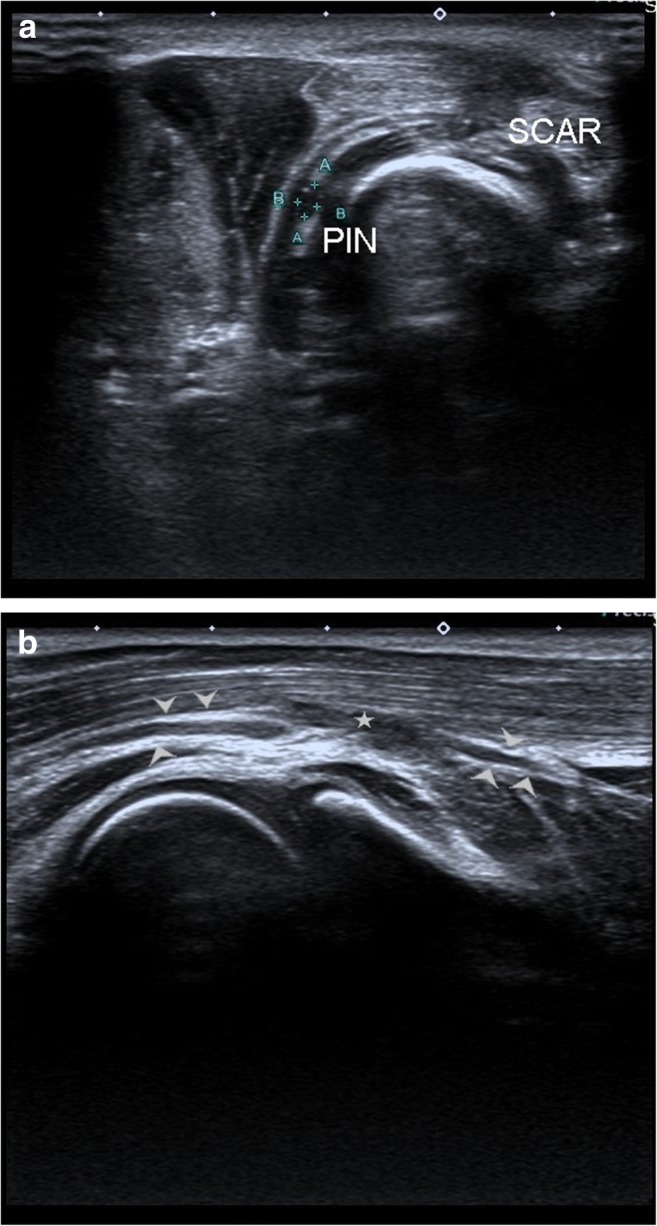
a High-resolution ultrasound image of a patient who had history of penetrating injury distal to the elbow. SCAR denotes the hypoechoic scar tissue formed within soft tissue at the site of injury. The posterior interosseous nerve (PIN) is normal at the level of local scar. b Image shows discontinuity in posterior interosseous nerve in the forearm with formation of hypoechoic scar tissue (stars), distal to the site of scar on skin. The nerve (arrowheads) is of normal calibre proximally and distally
